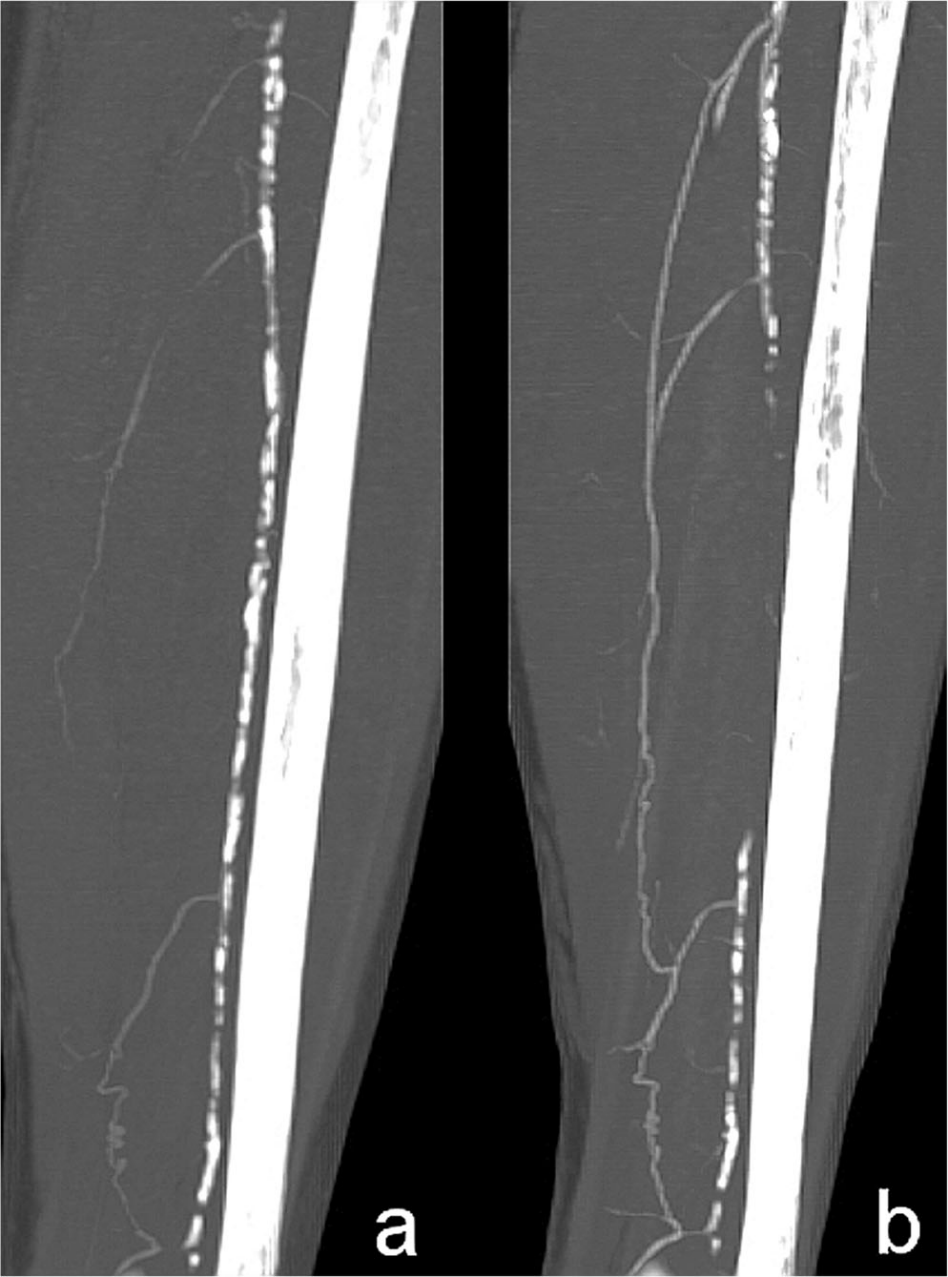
CTA angiograms – femoral longitudinal projection, shows the increase in length of collaterals; a: before plasmid administration; b: after 3 months from plasmid administration
